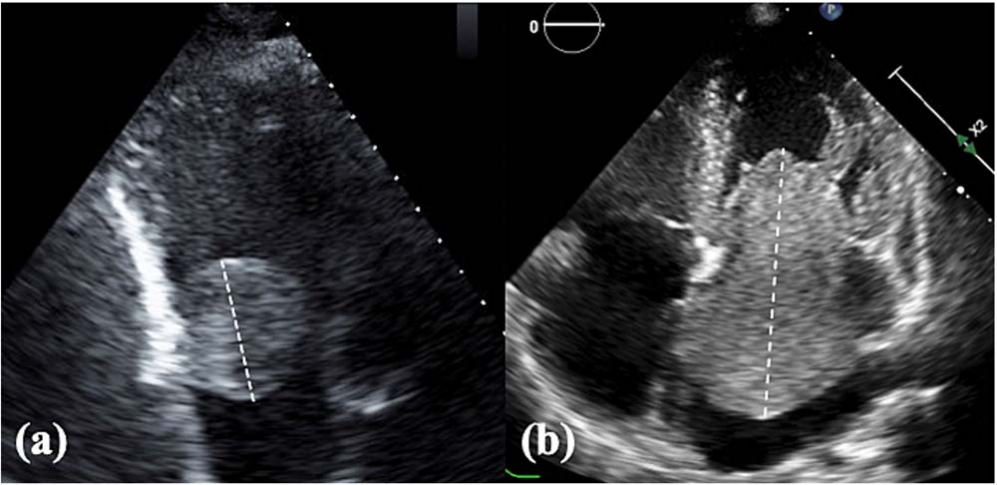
TTE findings. (a) Right atrial tumor and (b) left atrial tumor. Tumor size was assessed using the longest diameter measured by TTE. TTE, transthoracic echocardiography.
